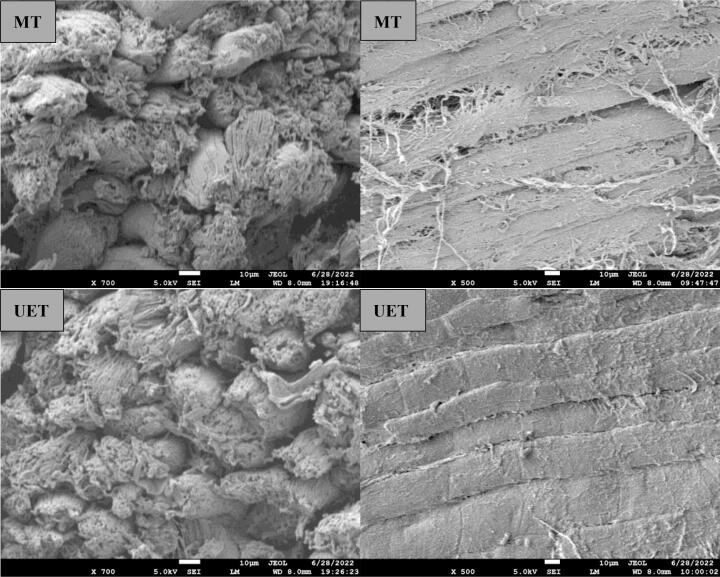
The cross-sectional and longitudinal microstructures of the samples under different methods. Control, fresh mutton; AT, air thawing; WT, water thawing; MT, microwave thawing; UET, ultrasound-assisted SAEW thawing.
